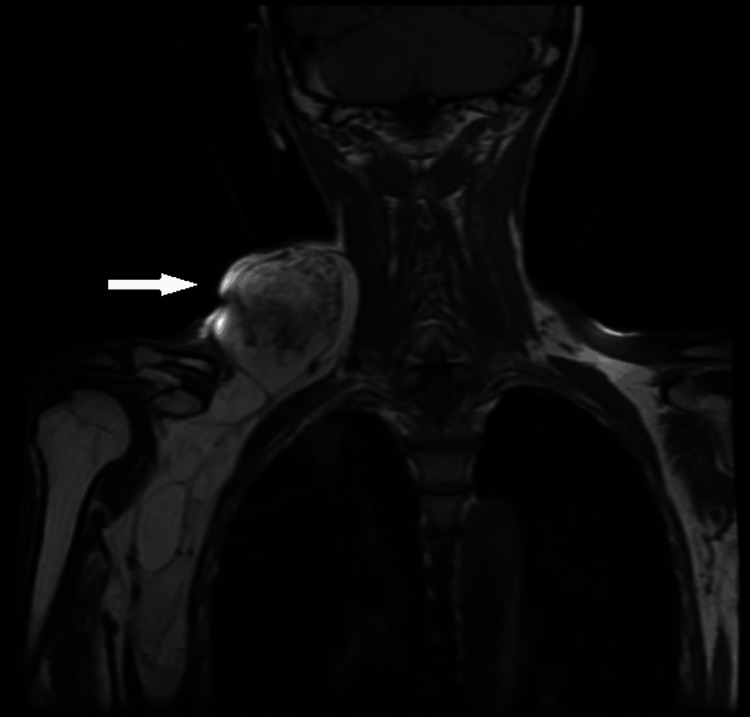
MRI showing space sarcoma in the cervicoaxillary canal
